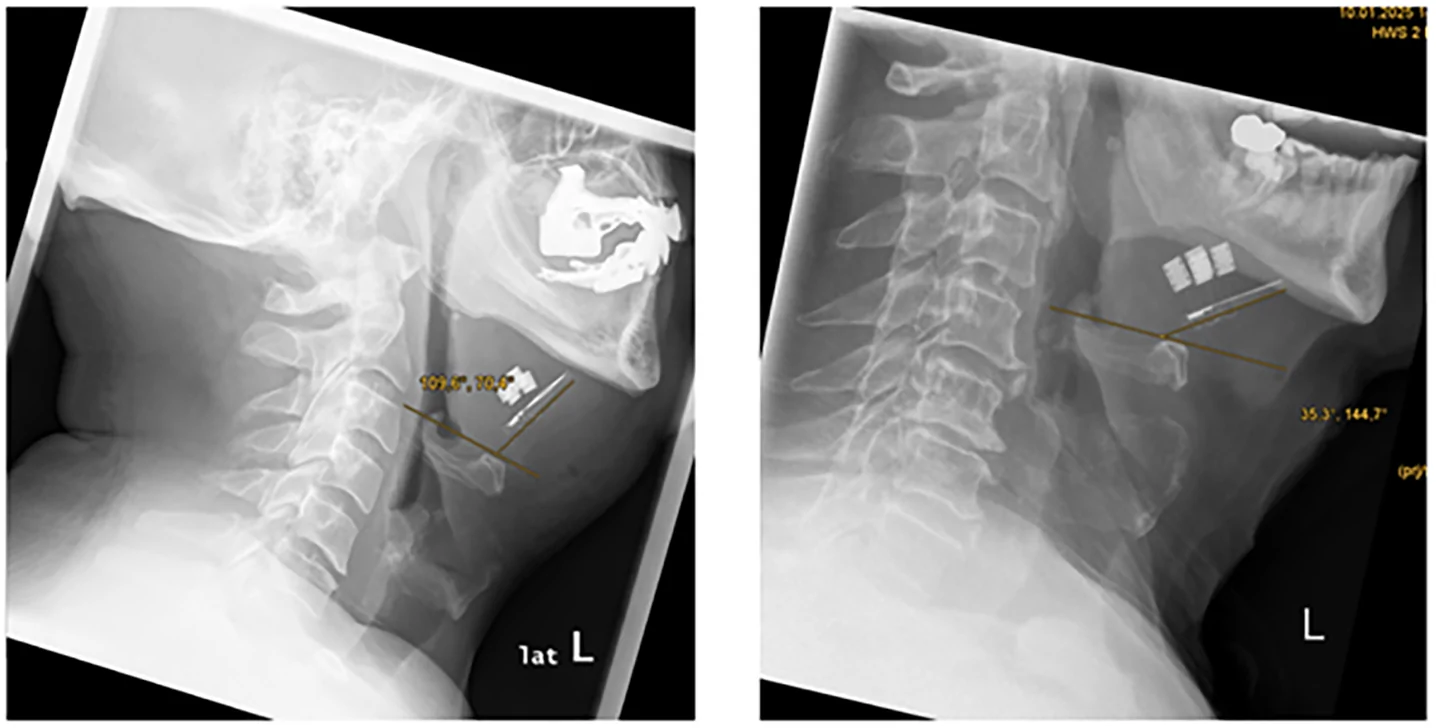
x-ray (lateral). Position of paddle electronics and angle of hyoid to implant (in°).
